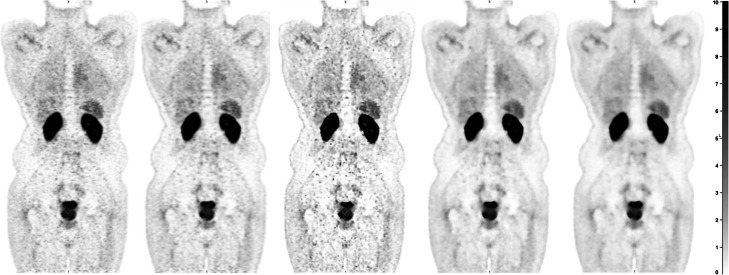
Coronal views of prostate cancer patient, scanned with ^68^Ga-PSMA with the data reconstructed using OSEM+TOF_6.4_, PSF_6.4_, BPL_200_, BPL_800_ and BPL_1200_ from left to right
